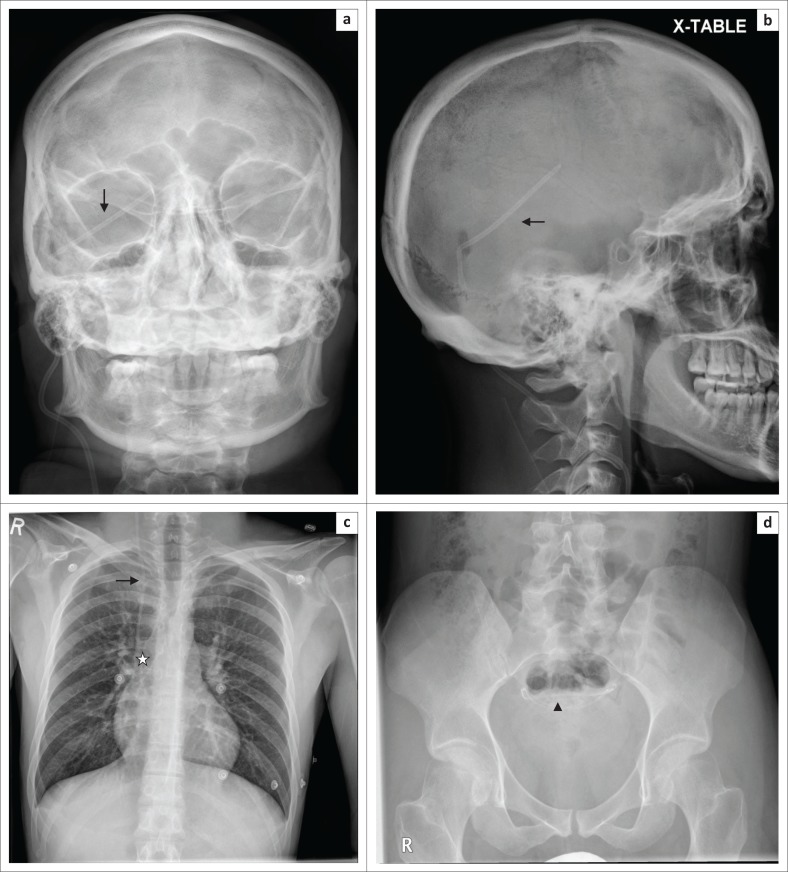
(a) Frontal radiograph of the head and neck of a typical shunt series showing the proximal catheter (arrow) in the right lateral ventricle; (b) lateral radiograph of the head and neck of a typical shunt series showing the proximal catheter (arrow) in the lateral ventricle; (c) chest radiograph of a typical (ventriculoatrial) shunt series showing the distal catheter (arrow) terminating at the cavoatrial junction (star) and (d) magnified image of an abdominal radiograph showing an abandoned ventriculoperitoneal shunt catheter in the pelvis (arrow).
